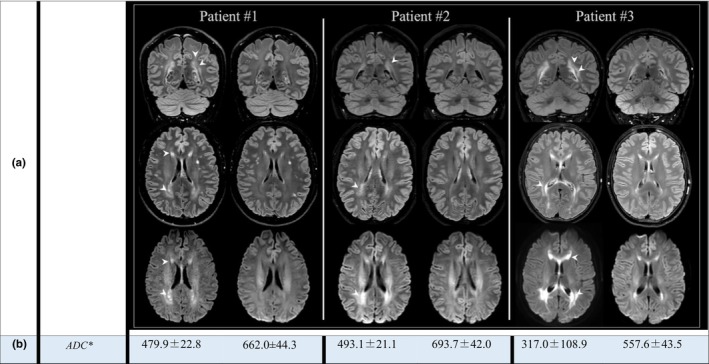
Brain magnetic resonance imaging coronal and axial fluid‐attenuated inversion recovery images and axial diffusion‐weighted images (a) and apparent diffusion coefficient (ADC) values (b) of three adult patients with phenylketonuria before treatment with pegvaliase (left column) and after phenylalanine stabilization (right column). Arrowheads indicate white matter abnormalities. *ADC values (mm^2^/s × 10^−6^); normal white matter ADC values are approximately 700 mm^2^/s × 10^−6^.
